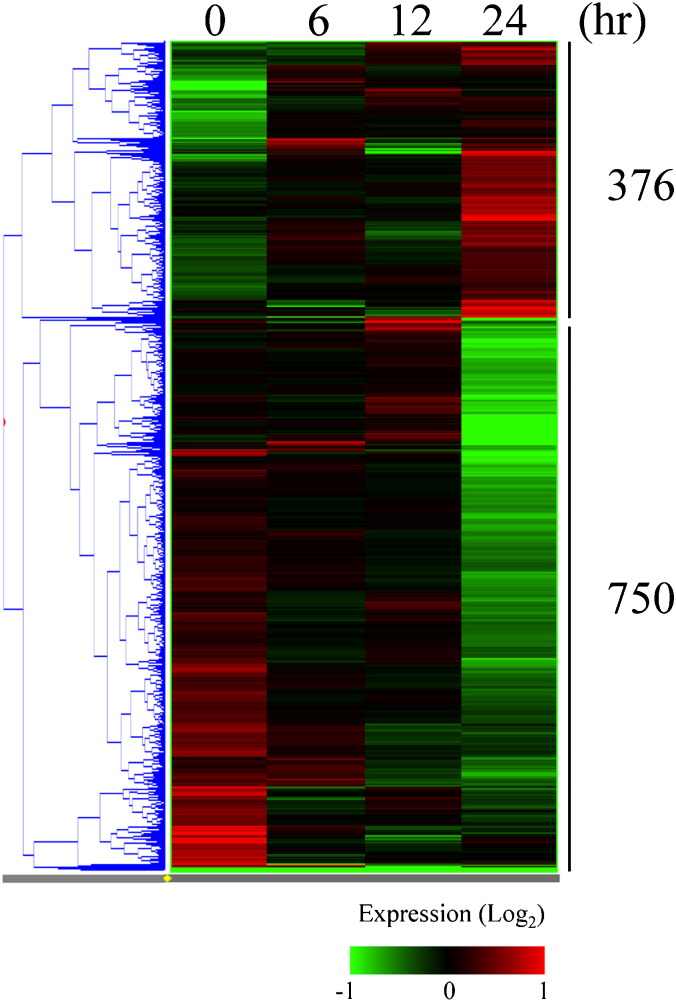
Gene expression profiles of non-irradiated and X-irradiated HUVECs. Genes that were differentially expressed by a factor of 1.5 or greater in HUVECs irradiated with 2.5 Gy X-ray are shown. Hierarchical clustering of differentially expressed genes was performed using the Gene Spring software.
